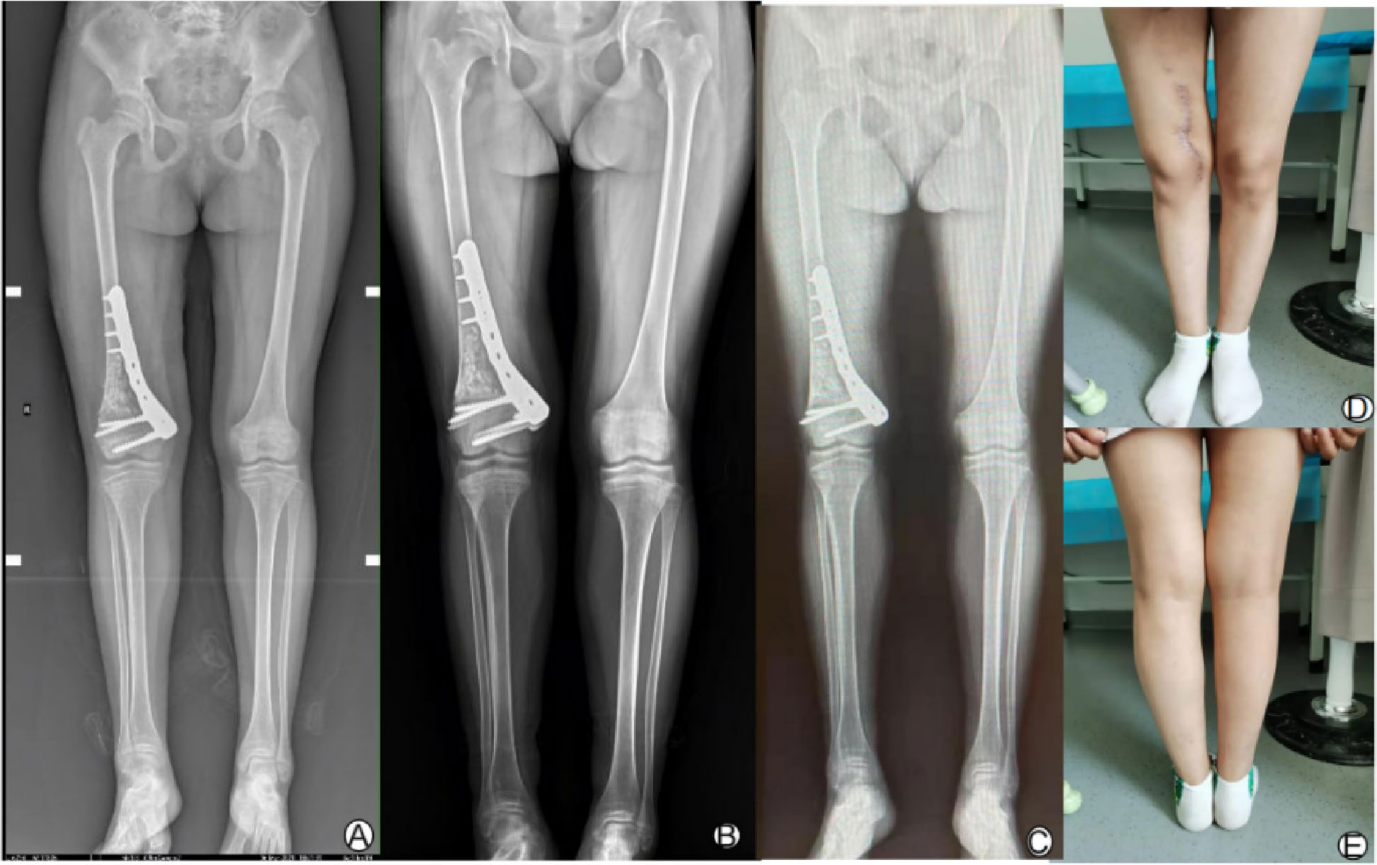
Ⓐ After the operation, the full-length X-ray showed that the mechanical axis of lower limb was corrected. Ⓑ Five months after the operation, X-ray showed that the lateral fracture line was blurred, the allogeneic bone particles in the medullary cavity were partially absorbed, and the mechanical axis of the lower limb was normal. No tumor recurrence was present. Ⓒ One year after the operation, X-ray showed that the lateral fracture line was completely healed, obvious osteogenesis was present in the medullary cavity, the mechanical axisof the lower limb was normal, and there was no tumor recurrence. Ⓓ-Ⓔ One year after the operation, and the mechanical axis of the lower limbs was normal by physical examination
